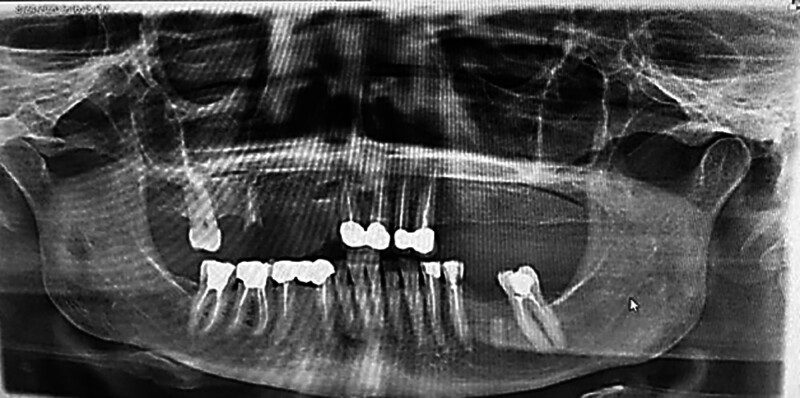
Radiographic representation of the lesion.
